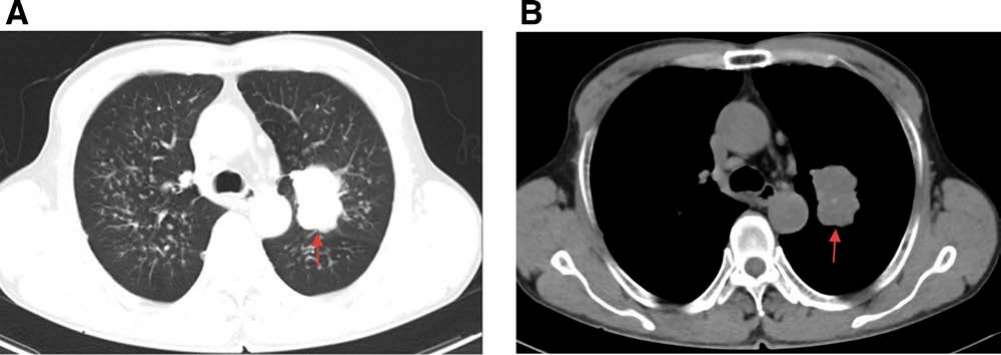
Axial CT images of the pulmonary (A) and mediastinal (B) windows reveal a soft tissue mass located in the left upper lobe of the patient’s lung. CT = computed tomography.
